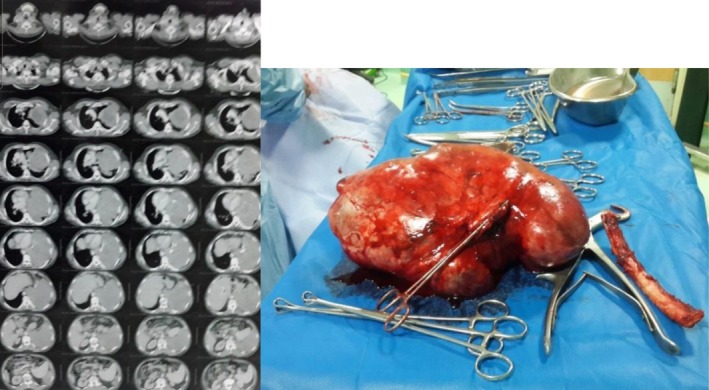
Left, lung CT scan shows a huge intrathoracic mass, which was extracted completely (right picture) by our method of surgery. The pathologic findings confirmed the diagnosis of a solitary fibrous tumor.
